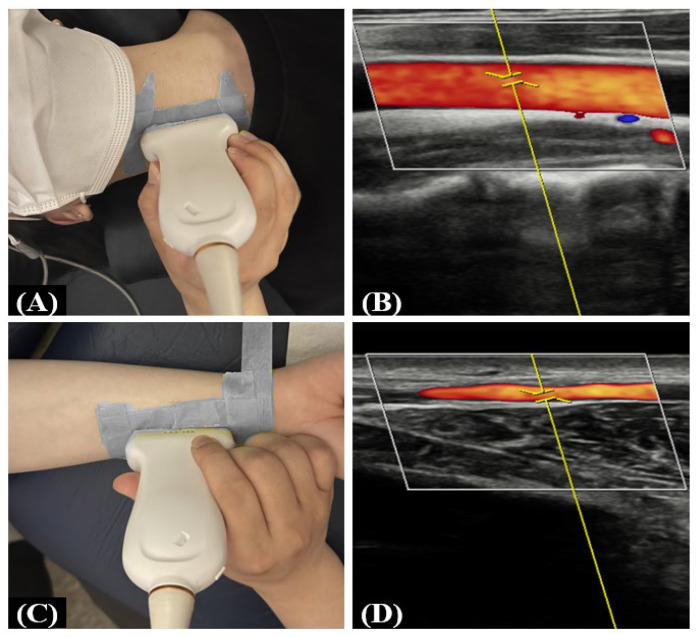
Probe positions for the CCA and RA and their Doppler images. (A,C) Probe positions for the CCA and RA, respectively. (B,D) Doppler images of the CCA and RA, respectively. The yellow line represents the angle of the Doppler effect in the Doppler mode of diagnostic ultrasound. The red and blue colors in the Doppler image represent blood flow into and out of the probe, respectively.
